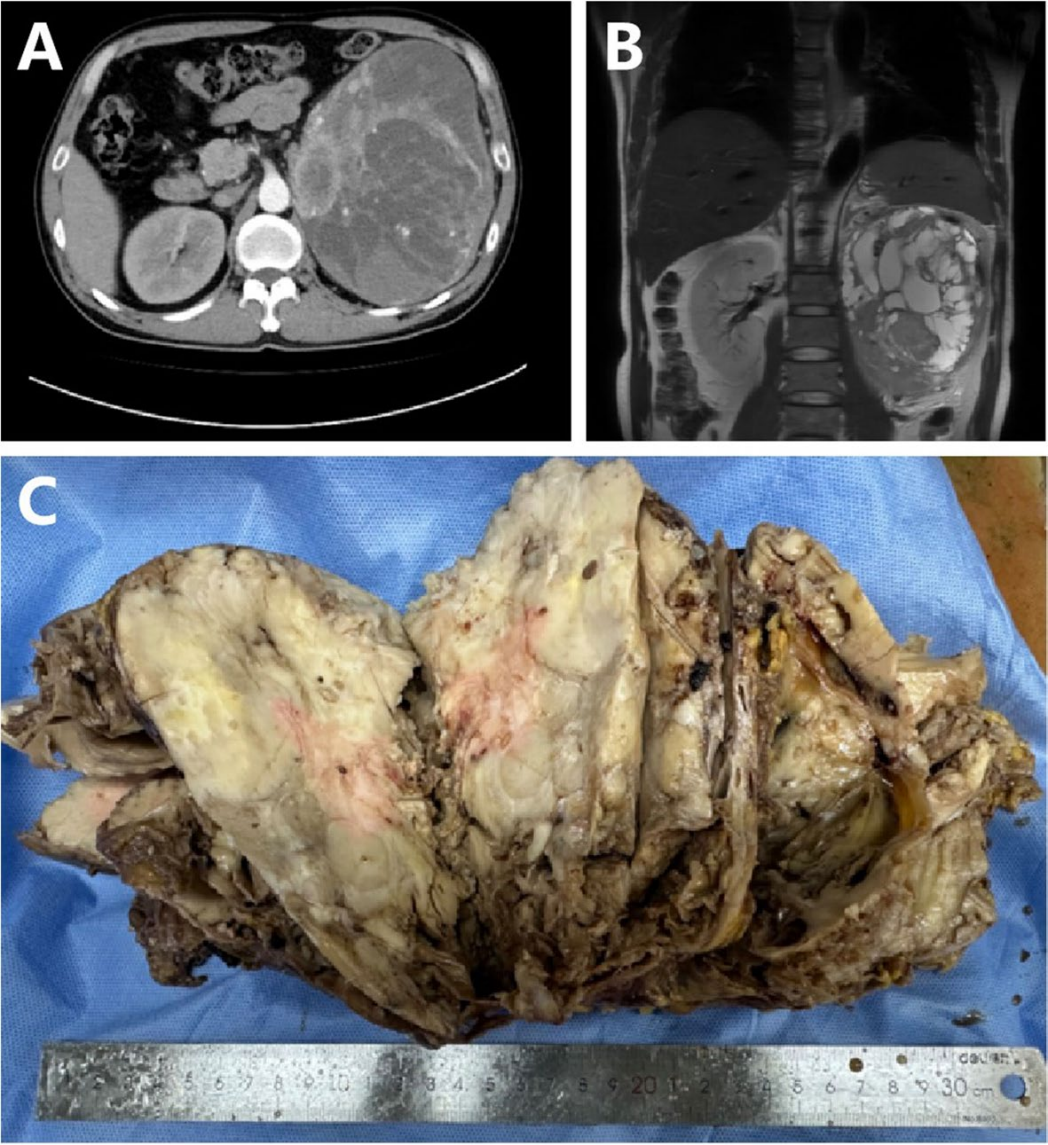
Computed tomography and magnetic resonance imaging showed a slightly enhanced, large, oval mass located at the upper pole of the left kidney (A and B). The tumor has a homogenously white and firm-cut surface (C)
